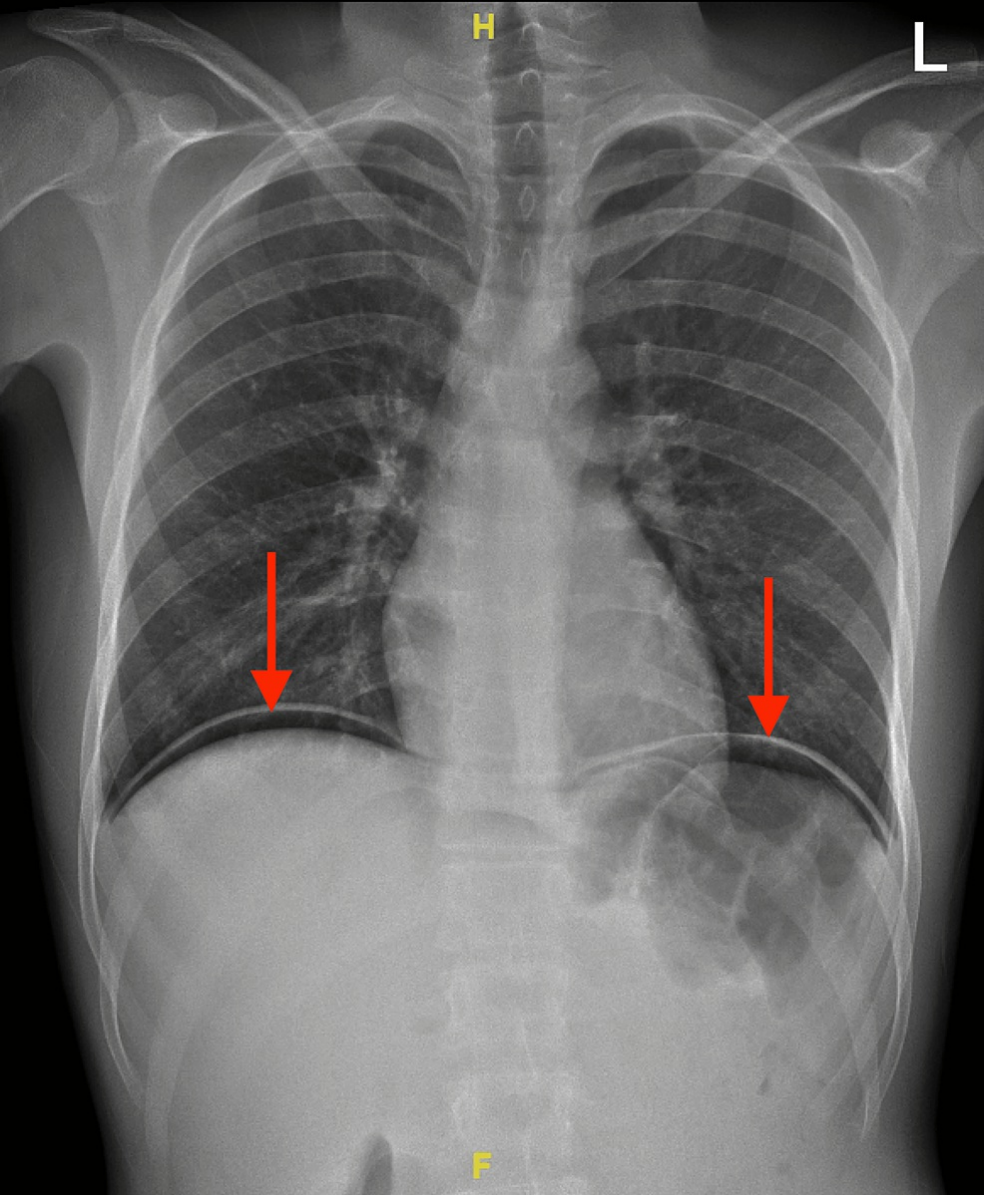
Posterior-anterior chest x-ray. The red arrow shows free gas under both diaphragmatic copula.
